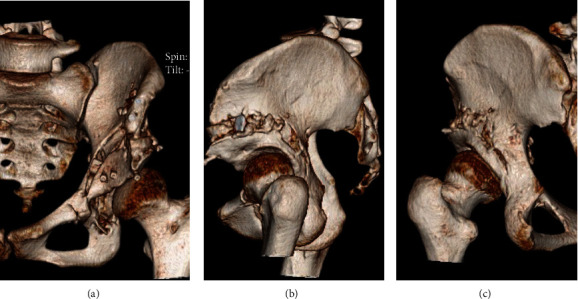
CT images taken one week after surgery: (a) frontal view, (b) sagittal view, and (c) posterior view. There was no fracture of the posterior column, and since the pubis was not completely osteotomized, there was no penetration of the osteotome into the joint, and the pubis was not completely cut. The pelvic ring has been preserved.
